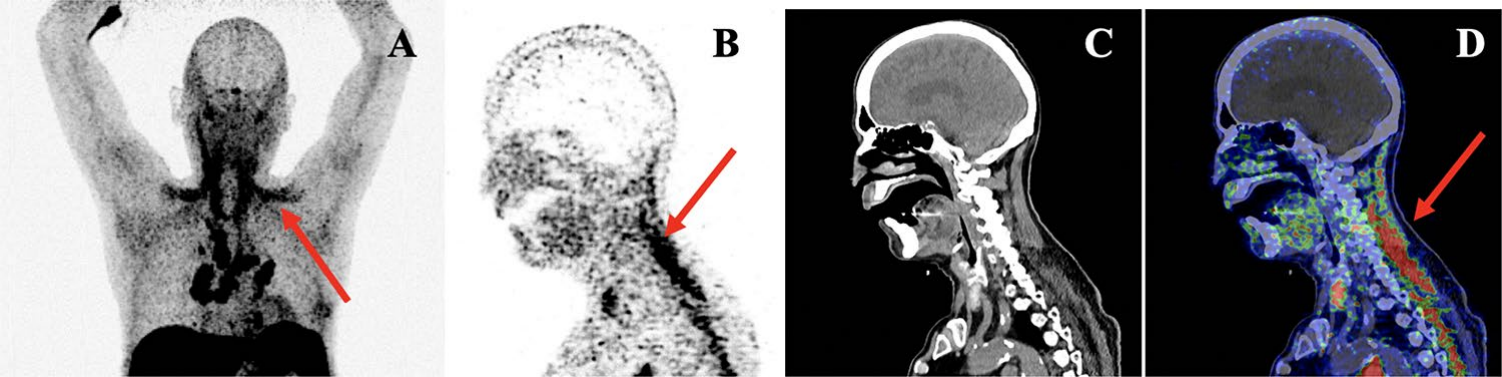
Signs of skeletal myositis on [^68^Ga]-DOTATOC PET/CT scan. Pathological uptake of the trapezius muscle (red arrows) (SUV scale 0–2); A Maximum intensity projection imaging. B Sagittal PET image, C Sagittal CT image and D Sagittal fused PET/CT image
